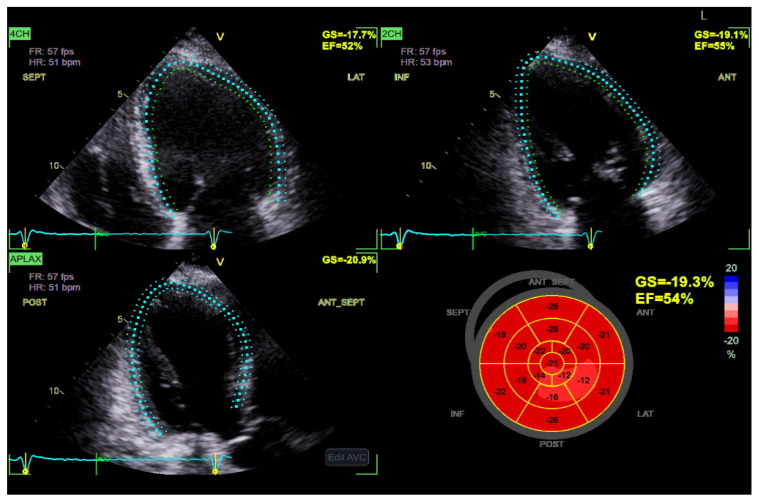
Global longitudinal strain (GLS) has increased sensitivity in diagnosing DBCM at its early stages and could be a useful index for the follow-up in patients with T2DM. A progressive decrease in GLS, in serial echocardiographic studies of the same patient, may serve as an early marker of emerging diabetic cardiomyopathy. (Original images from authors’ institutions).
